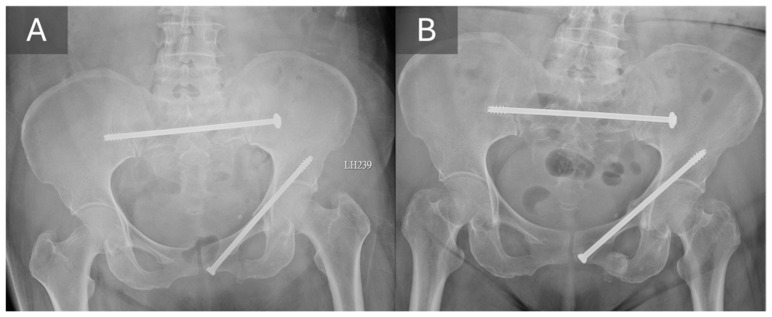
A typical case of using TITS screw in posterior ring fixation. Comparing the radiograph obtained immediate after operation (A) with the 6-month follow-up radiograph (B), the alignment was well maintained, and no screw loosening was noted.
